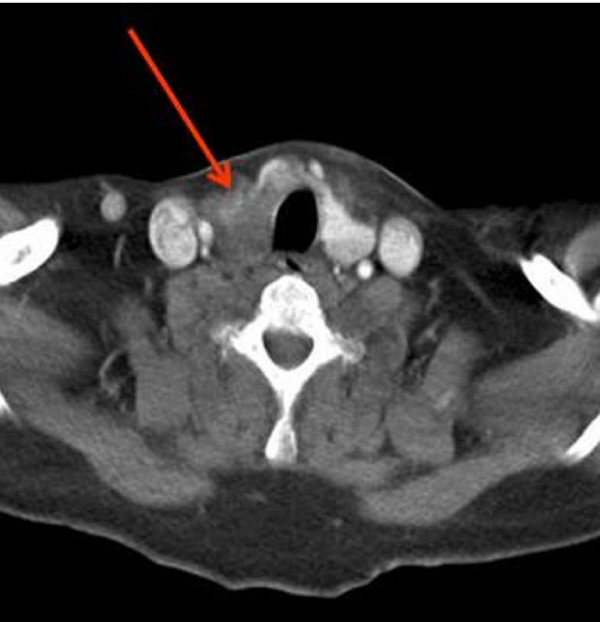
Computed tomography (CT) scan of the neck and thoracic outlet. Repeat CT scanning after ten months of steroid therapy confirms reduction in size of the goitre and with return of the trachea to midline position.
